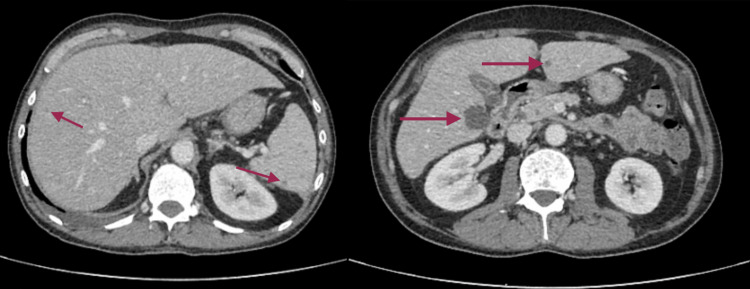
Abdominal CT-scan showing multiple hepatic and splenic nodules suggestive of infeccious foci Abdominal ultrasound showing splenic nodules that were first thought to be infection but later found to be metastases, after biopsy of one of the hepatic lesions.
